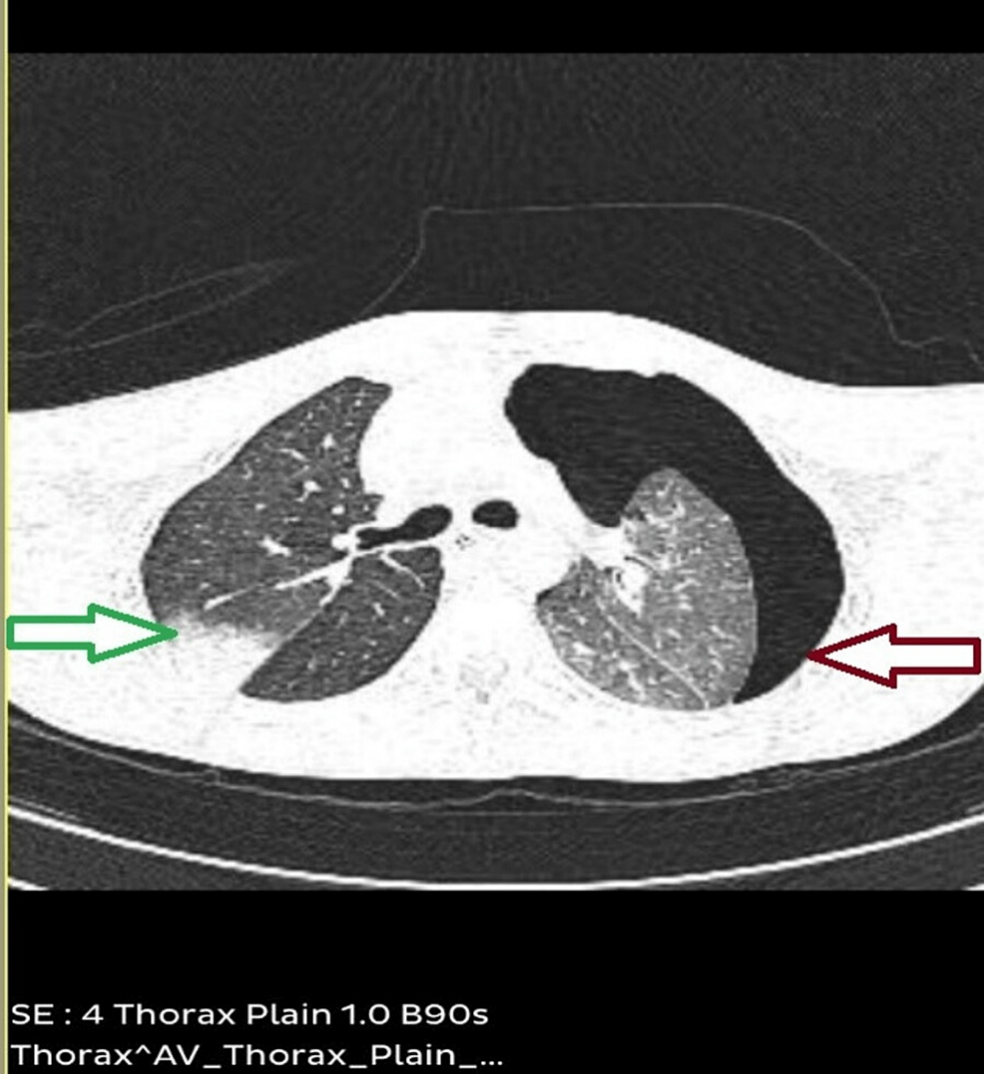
HRCT of the thorax showing consolidation on the left side (green arrow) and pneumothorax on the right side (red arrow)
